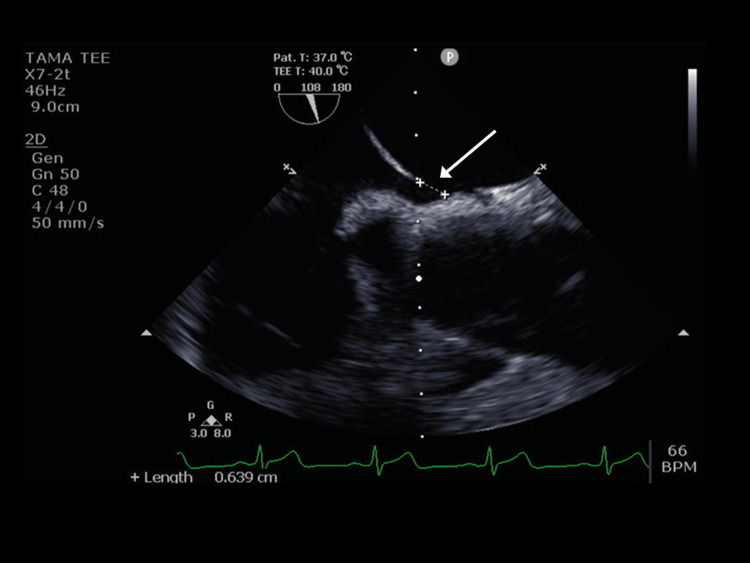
Transesophageal echocardiography reveals a 5-6-mm foramen ovale (arrow).
